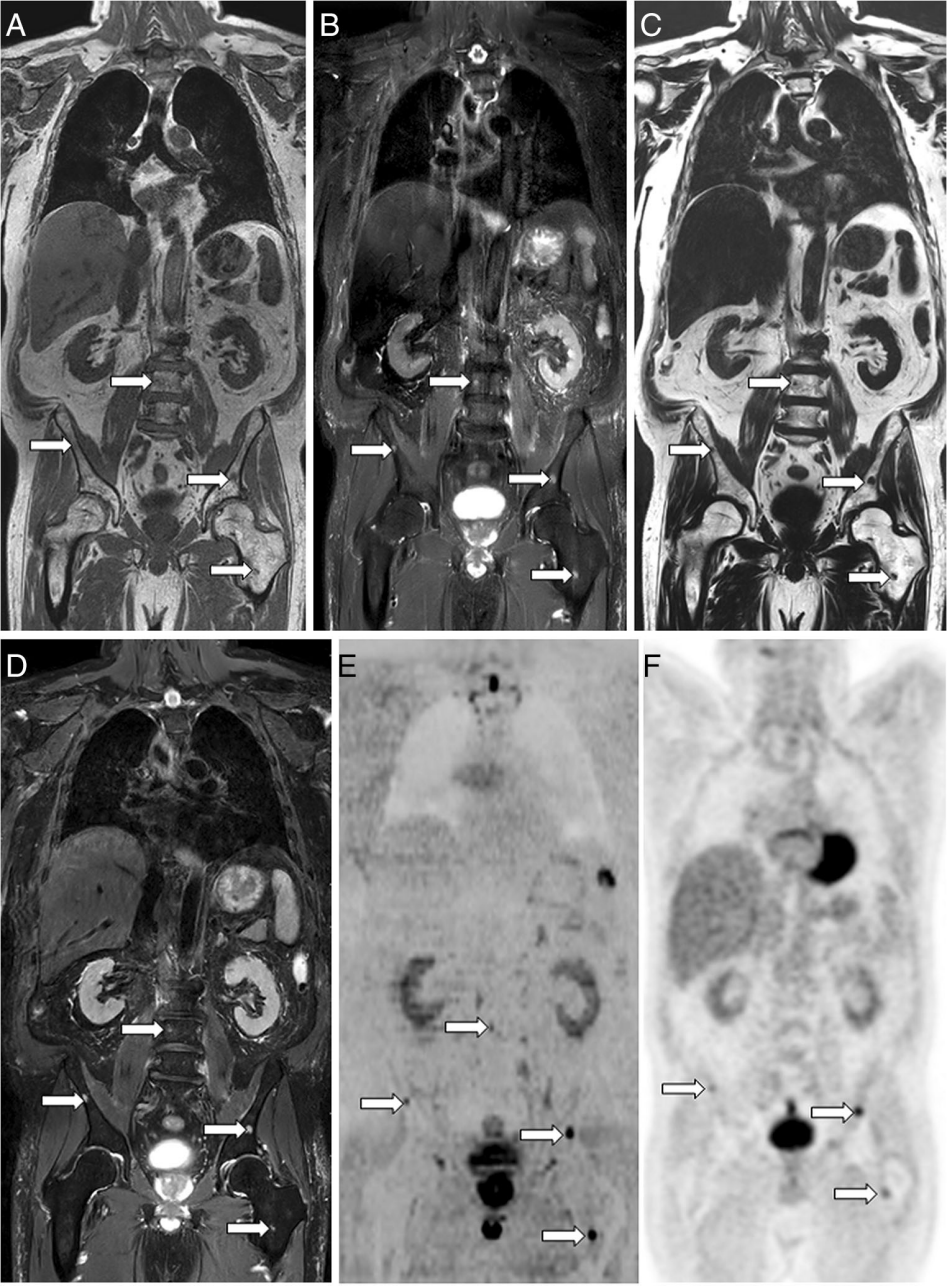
Agreement between sequences on myeloma lesions: WB-MRI study in a 65-year-old man with newly diagnosed multiple myeloma. Several lesions are observed in the lumbar spine, iliac bones, and left femur (arrows). Coronal T1 (A) and STIR (B) WB-MRI images show four lesions. Fat (C) and water (D) reconstructions of the TSE T2 Dixon acquisition show the same lesions. The reference standard, based on reading of all MR images and concurrent imaging studies, confirmed the presence of these lesions. E Concurrent DWI image (*b* = 1000 s mm^−2^, inverted gray scale) confirms the presence of lesions. F [^18^F]F-FDG PET/CT image shows iliac and left femur lesions presenting tracer uptake
